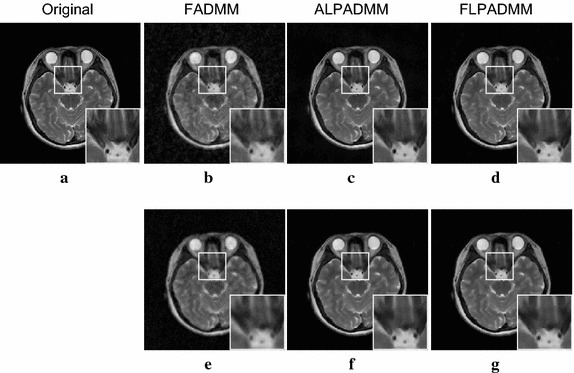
Reconstructed images and zoomed-in regions among the state-of-the-art MR image reconstruction algorithms using a pseudo-Gaussian mask (*first row*) and pseudo-radial mask (*second row*) with 25% sampling. a Original human brain1 image, b, e FADMM, c, f ALPADMM, d, g FLPADMM
